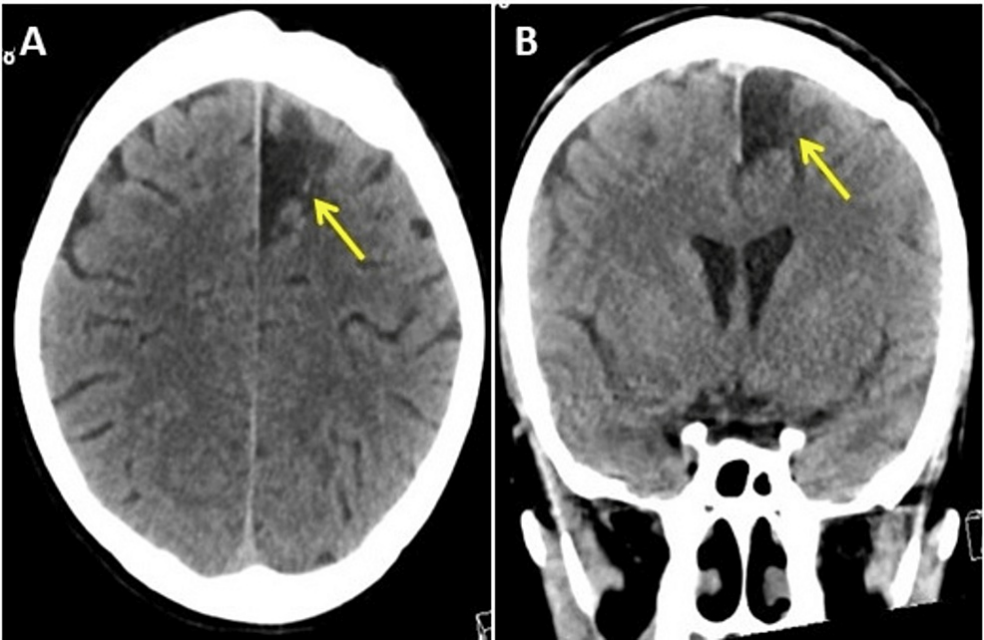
Follow-up computed tomography (CT) scan images of the brain two years after the surgery. Follow-up CT scan two years after surgery, panel (A) axial view, and panel (B) coronal view of the brain showing focal cortical volume loss and encephalomalacia (yellow arrows) at the surgical bed with complete resolution of the edema and mass effect.
